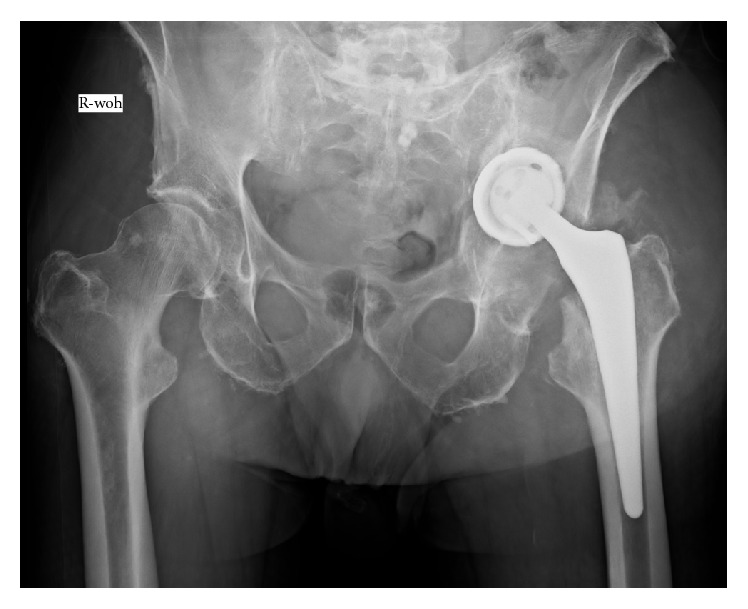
Radiograph of pelvis (deep) after fall: periprosthetic acetabular fracture (type IIIa acetabular defect according to Paprosky et al. [20]).
